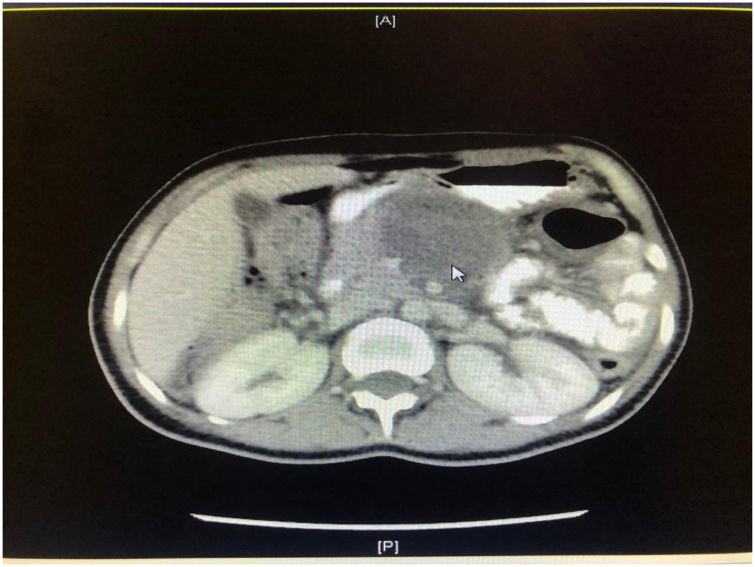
Axial enhanced computed tomography scan of the abdomen showing an obstructing transverse colon mass (arrow).
